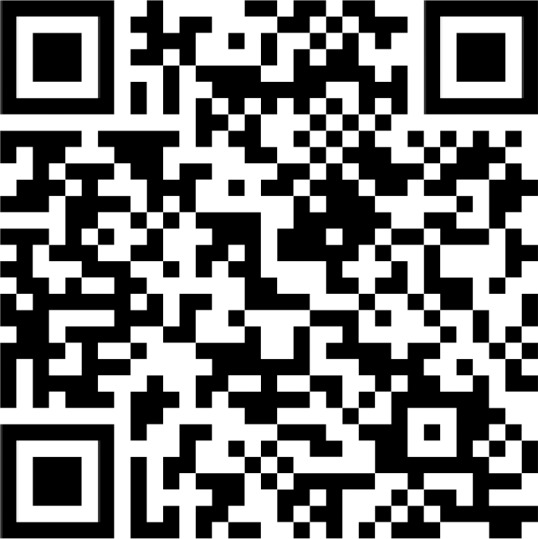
Transesophageal echocardiography showing multiloculated hydatid cyst within the interventricular septum.
